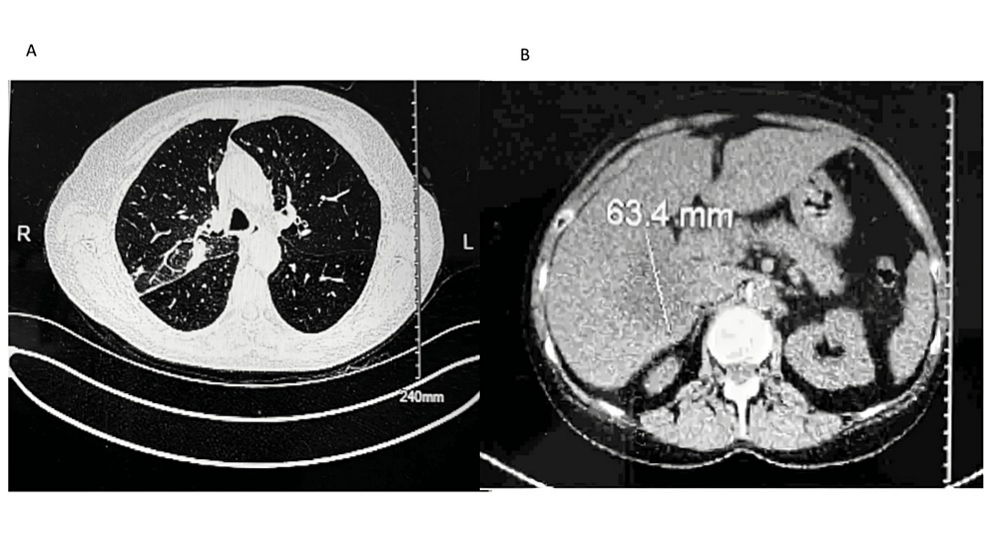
The CT chest without contrast showed right lung consolidation or mass (2A), and hepatic mass (2B).
